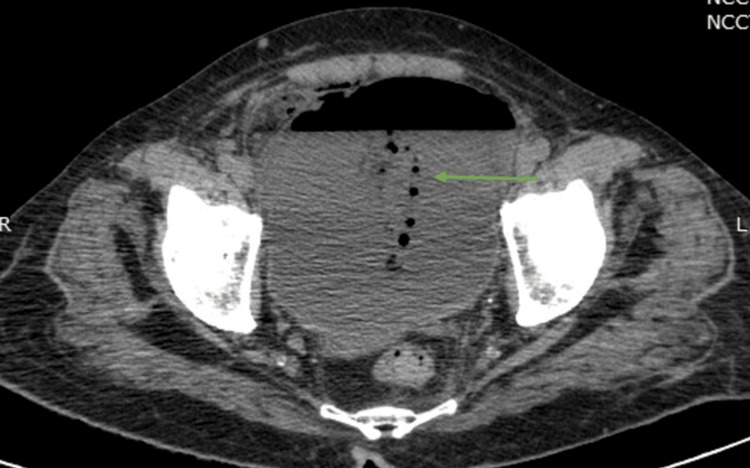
Follow-up CT KUB performed at four weeks, demonstrating persistent intramural gas within the bladder wall and minimal reduction in the perivesical inflammatory collection (arrow). CT KUB: Computed tomography of the kidney, ureter, and bladder.
